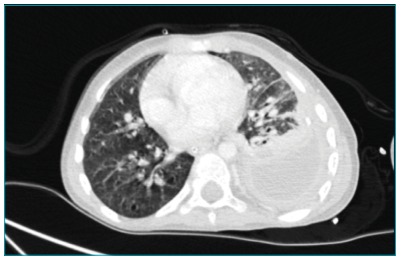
Thoracic computed tomography scan performed on admission to a
